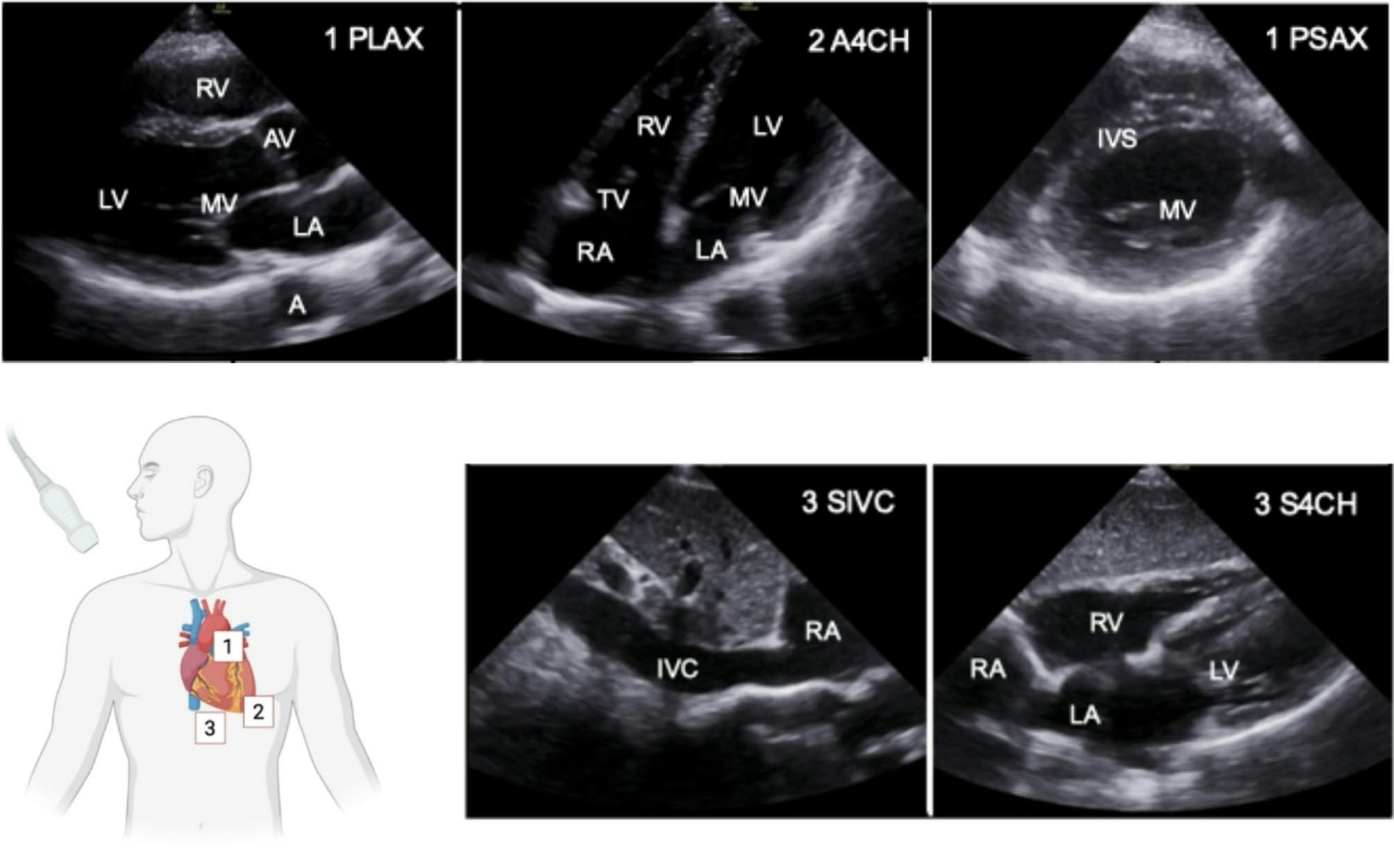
Basic FoCUS examination views. Prehospital TTE can be performed using the parasternal window (1) to assess the parasternal long-axis (PLAX) and short-axis (PSAX), the apical 4-chamber-view (A4CH) (2) and the subcostal window (3) for the subcostal inferior vena cava (SIVC) and subcostal 4-chamber (S4CH) [56]. A = aorta, AV = aortic valve, IVC = inferior vena cava, IVS = interventricular septum, LA = left atrium, LV = left ventricle, MV = mitral valve, RA = right atrium, RV = right ventricle, TV = tricuspid valve. Created with BioRender.com
